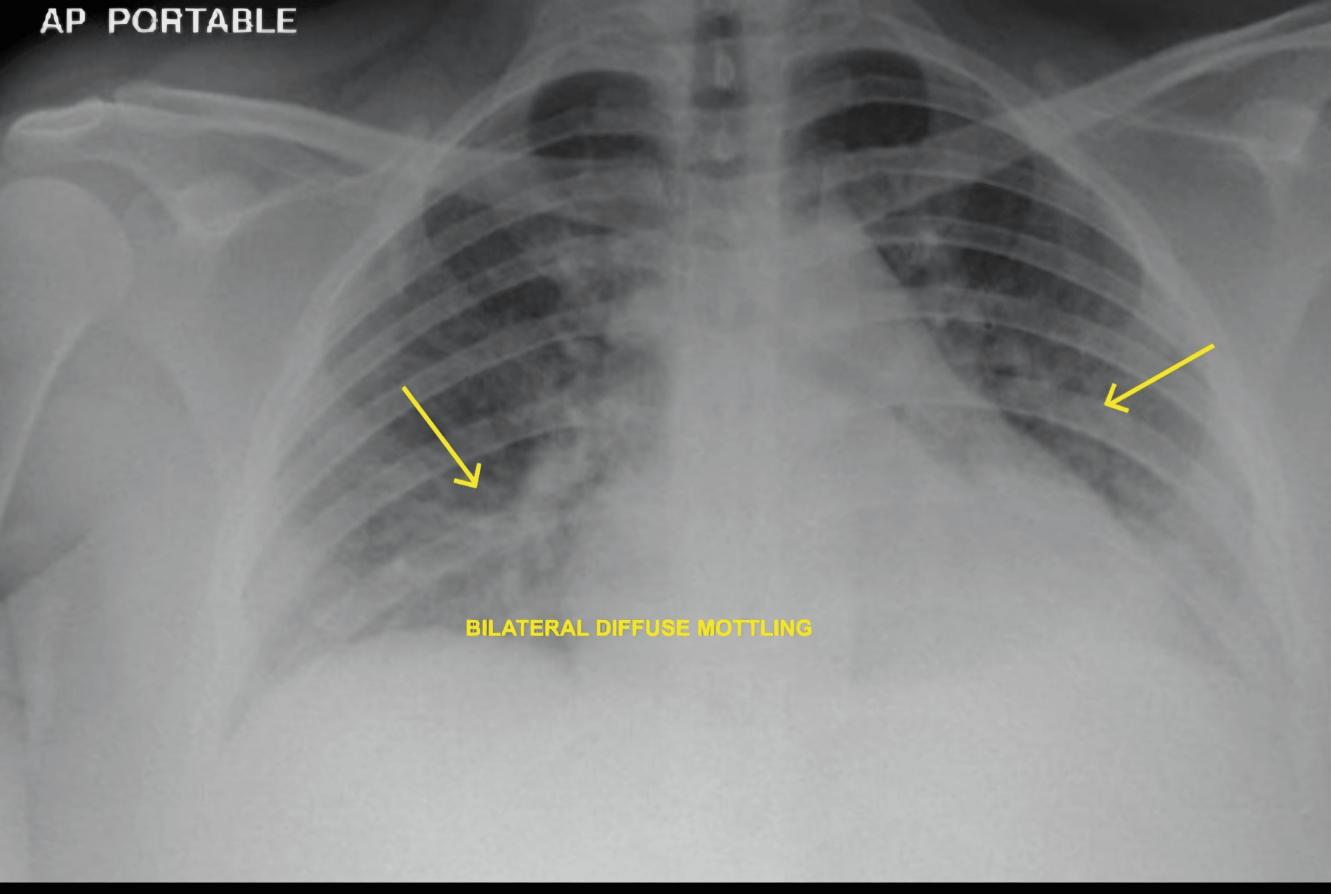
Chest X-ray showing bilateral diffuse mottling suggestive of bronchopneumonia
